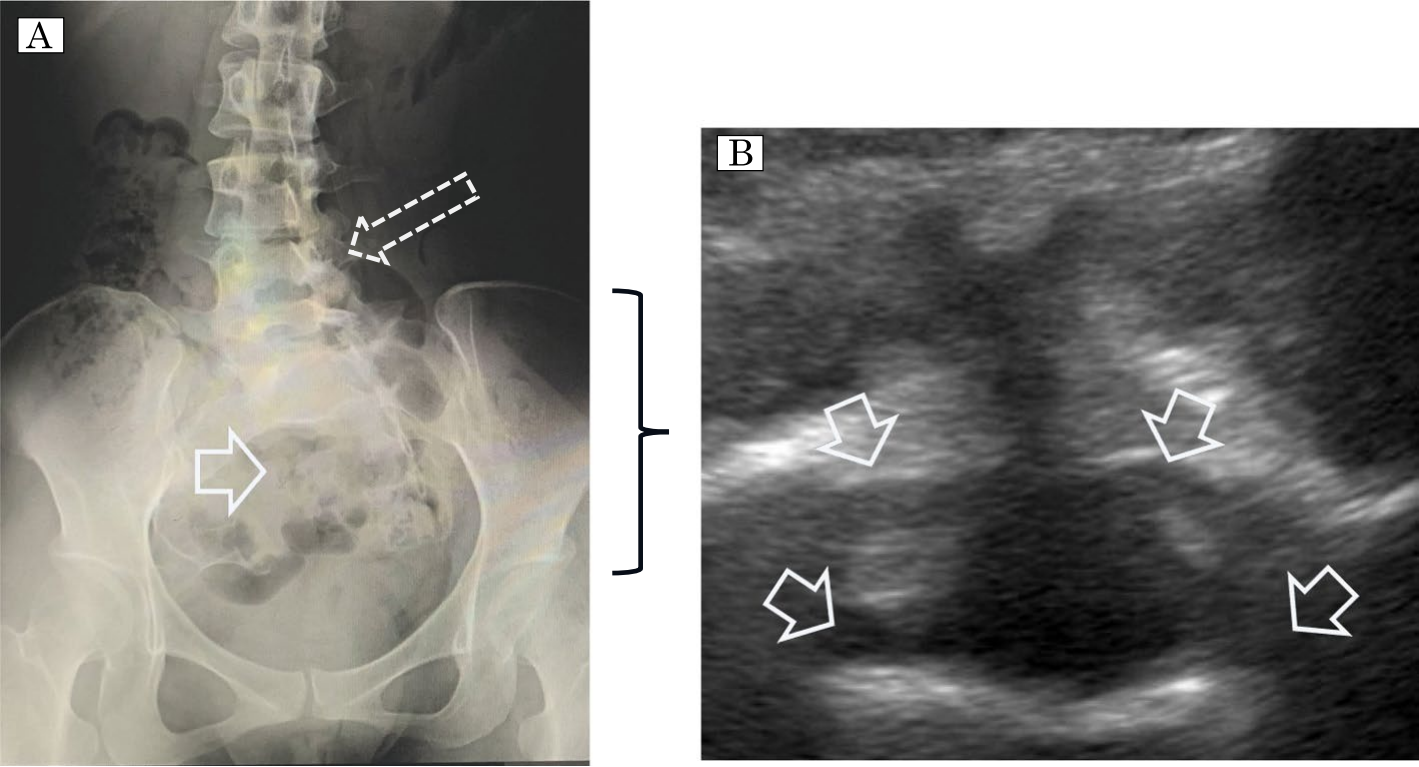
A Radiographical image of the lumbar spine on AP view. A solid arrow shows incomplete fusion and a bone defect. The dashed arrow shows scoliosis and rotation from the lower lumbar vertebrae to the sacrum. B Ultrasonographic image of axial view at the level of L5. Incomplete fusion of the vertebra at L5 is indicated. Solid arrows showed the incomplete fusion of the vertebra at L5, hypoplasia in the spinous process, and hypoplasia in the vertebral arch of the lumbar spine, similar to the radiographic findings
